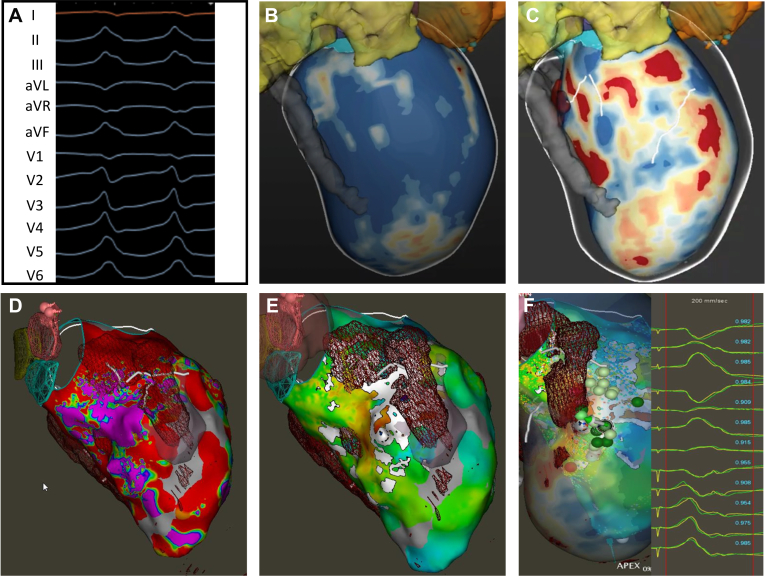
Target delineation in a case with agreement of imaging-based scar and electrophysiological data. A: Ventricular tachycardia (left bundle, inferior axis with V2 precordial transition suggestive of left ventricular outflow tract origin) induced during noninvasive electrophysiological study using s3 protocol. B and C: Cardiac magnetic resonance image processing using ADAS software showing healthy tissue in the epicardial aspect (*blue color*) and scar (*red*) in the endocardial aspect. The scar-mediated ventricular tachycardia channel is identified as a *white line* between 2 scar (*red*) areas. There is an endocardial scar in the midanteroseptal area. D: Endocardial bipolar voltage with presence of scar (*red*) in the basal to midanteroseptal area. *Purple area* identifies healthy voltage. E: The *brown mesh* identifies the magnetic resonance imaging–based scar, and the *white area* identifies the presence of wavefront discontinuity lines in an activation map obtained in sinus rhythm. F: Pace mapping at the areas of interest. The pace-map agreement of >95% labeled as *dark green* and >90% as *light green dots*.
